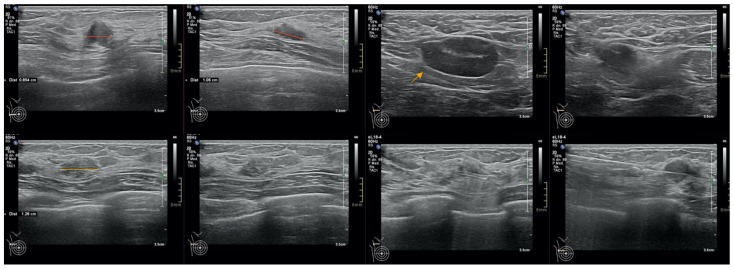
Ultrasonographic image showing irregular, solid, hypoechoic nodules (red lines), suspicious for malignancy. Axillary lymph nodes are also visible (indicated by orange arrows and lines), some of which display altered morphological features.
